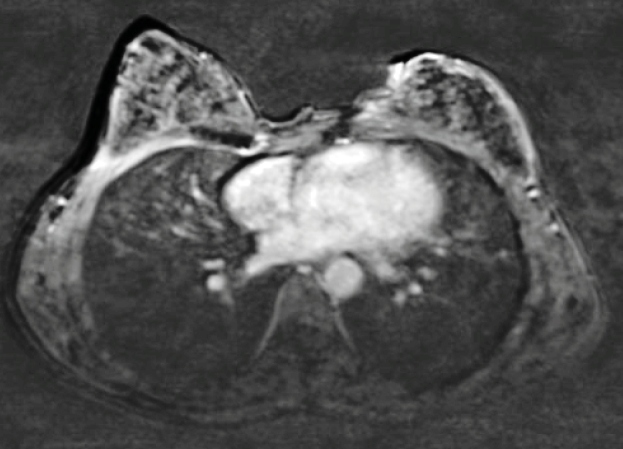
Axial T1 weighted MRI image: Post-treatment axial contrast-enhanced image shows no inflammation and/or abscess at the left breast.
